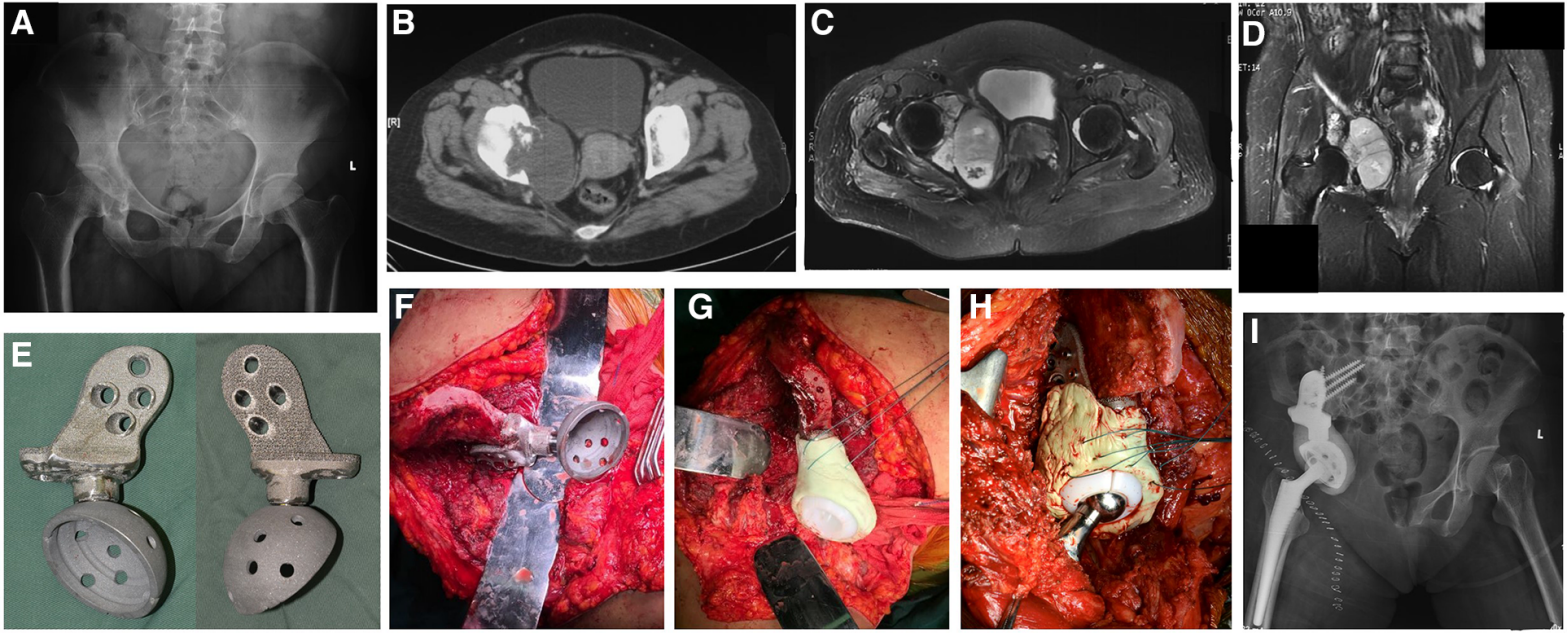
Periacetabular tumor resection and prosthetic reconstruction. Preoperative x-ray (A), CT (B) and MRI (C,D) images showing Zone II + III chondrosarcoma patients with periacetabular involvement. (E) Anterior and posterior images showing the design of a hemipelvic prosthesis with a 3D printing interface mimicking trabecular bone. (F–H) Intraoperative image showing fixing the hemipelvic prothesis to the remaining ilium, attaching the polyethylene bushing to the prothesis with bone cement, and reducing the hip with a total hip arthroplasty component from the proximal femur. (I) Postoperative x-ray.
